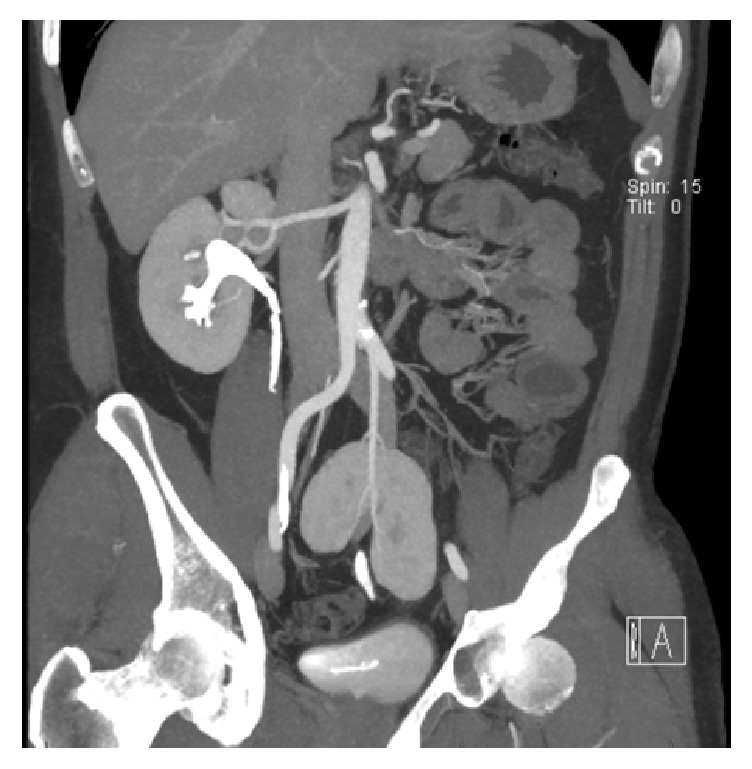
CT scan with coronal image of ectopic left pelvic kidney showing the middle polar artery.
